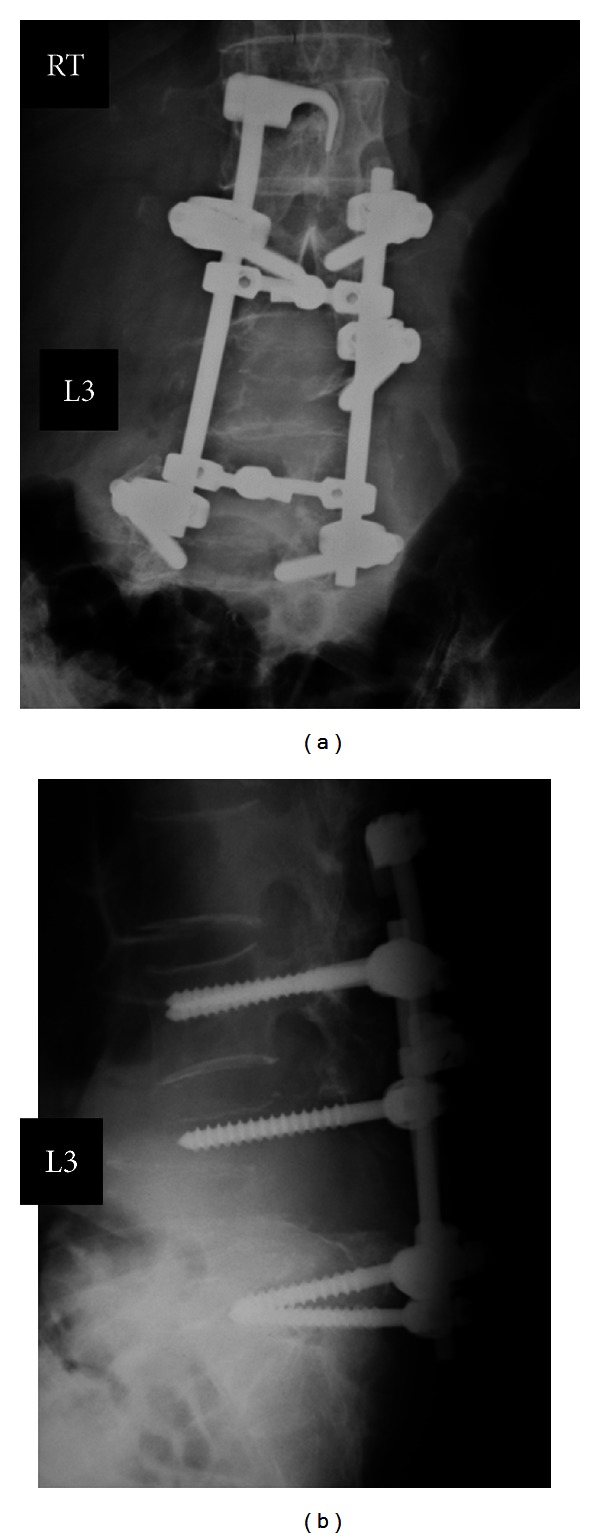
Plain radiographs before the second surgery (February 2010) show that the pedicle screws inserted at L3 and L5 had completely loosened and were displaced.
